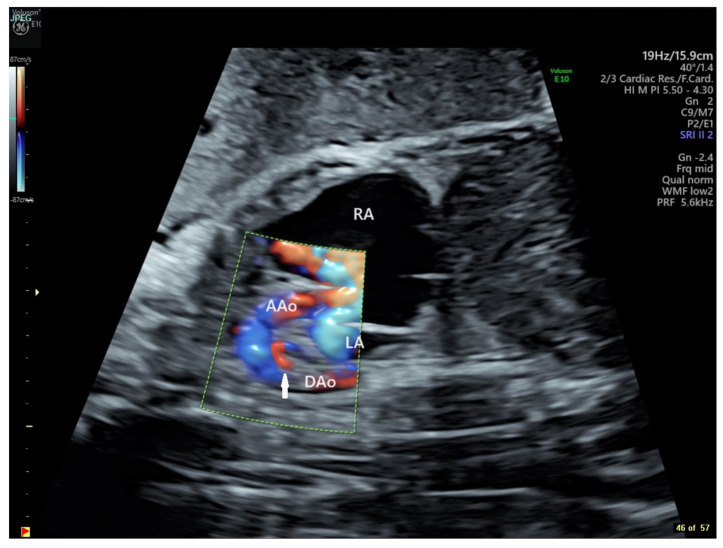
Echocardiographic sagittal still frame image of the fetal thorax with color flow mapping demonstrating retrograde flow in a diminutive ductus arteriosus (arrow). RA = Right Atrium, AAo = Ascending Aorta, LA = Left Atrium, DAo = Descending Aorta (GE Voluson E10 Imaging System).
